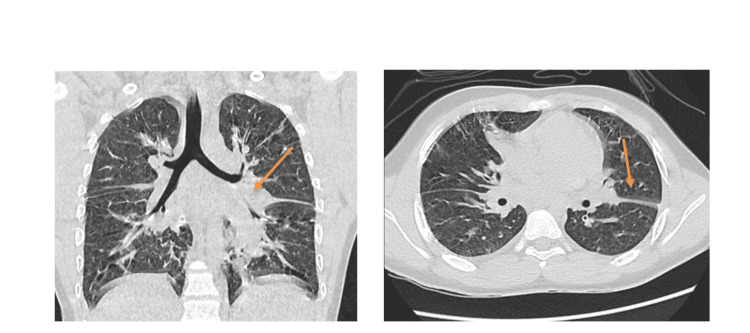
High-Resolution CT (HRCT) coronal and axial views showing bilateral ground glass appearance with septal thickening; patchy consolidation in the right middle lobe and in both lower lobes
